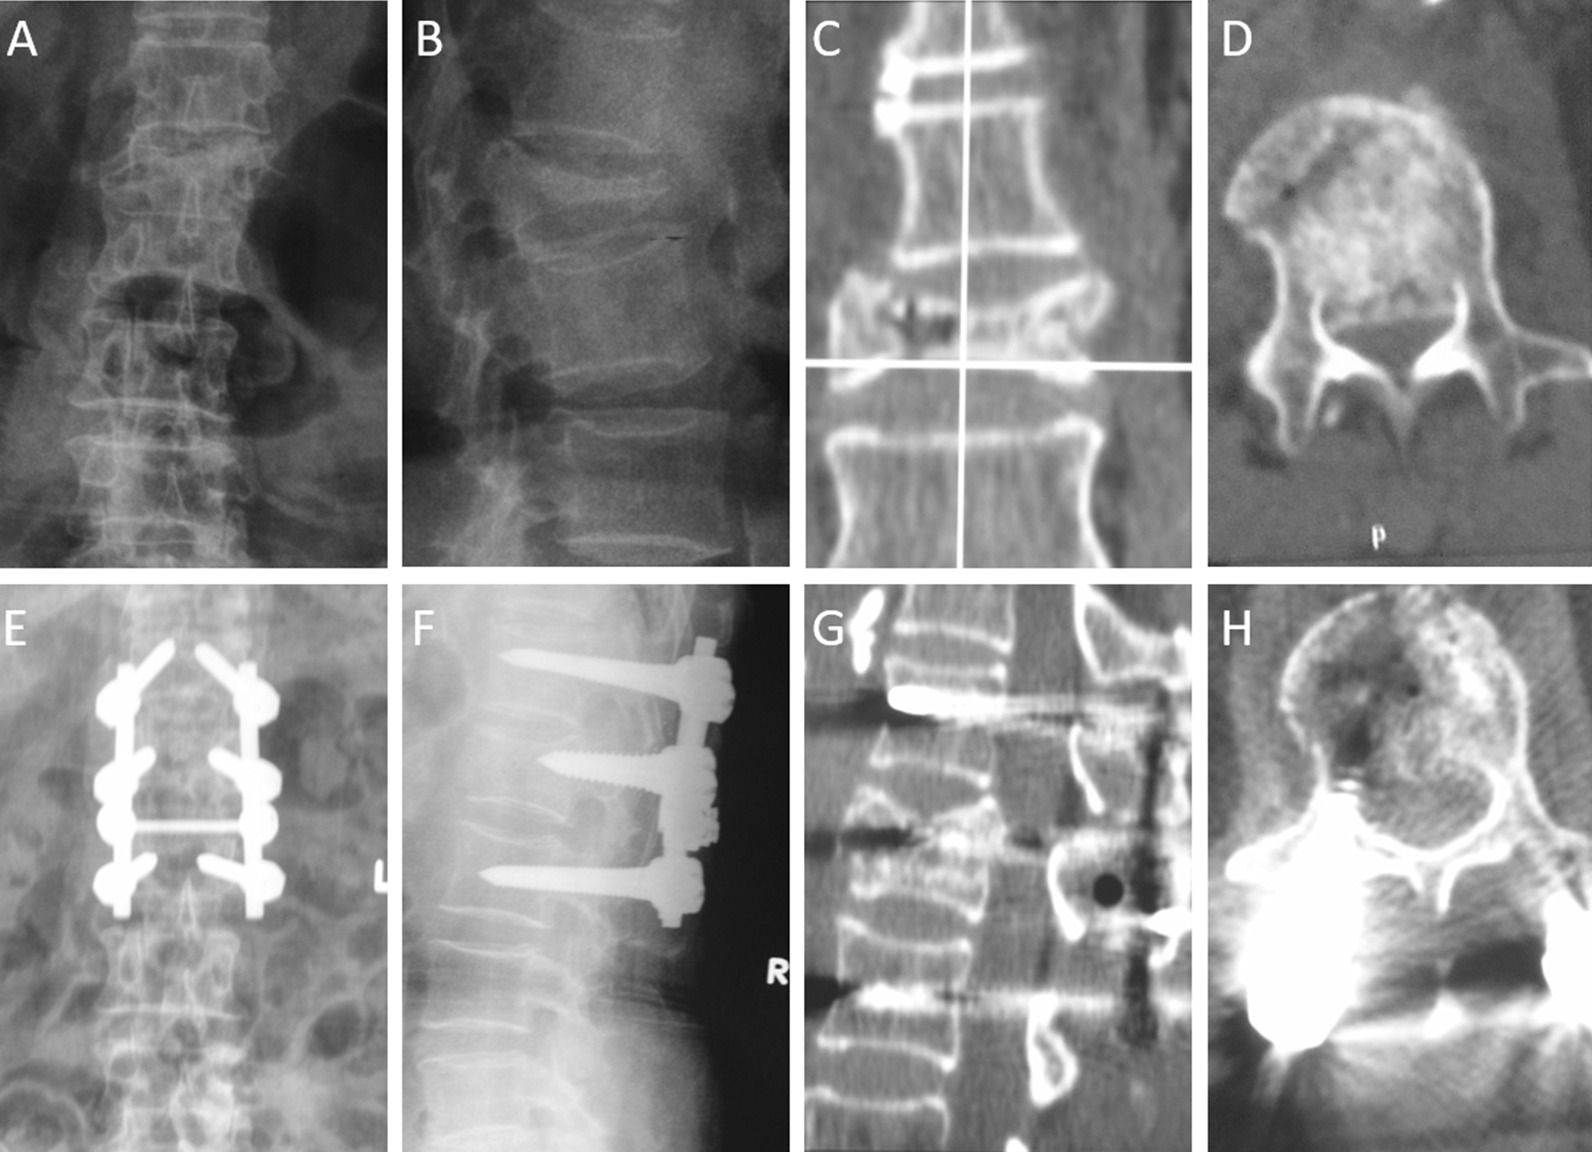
A 61-year-old female patient with back pain, AO type B burst fracture of Lumbar 1, and ASIA grade B paralysis due to fall injury. The preoperative anteroposterior X-ray view a and lateral X-ray view b showed the collapsed, burst and wedge-shaped L1 vertebra. The interpedicular distance was widened and the pedicle was deformed. The preoperative coronal CT c and axial CT d showed the decreased height of the vertebral body, the longitudinal fissure, vacuum phenomenon, and spinal canal space occupyed by burst fracture fragment. The 2nd day postoperative anteroposterior e and lateral X-ray f view showed a good position of the instrumentation, well reduced L1 vertebra with good sagittal alignment and height. The Sagittal CT image g showed the height of the vertebral body has been expanded and restored. Axial CT image h showed fracture fragment was reduced with good restore of the spinal canal
